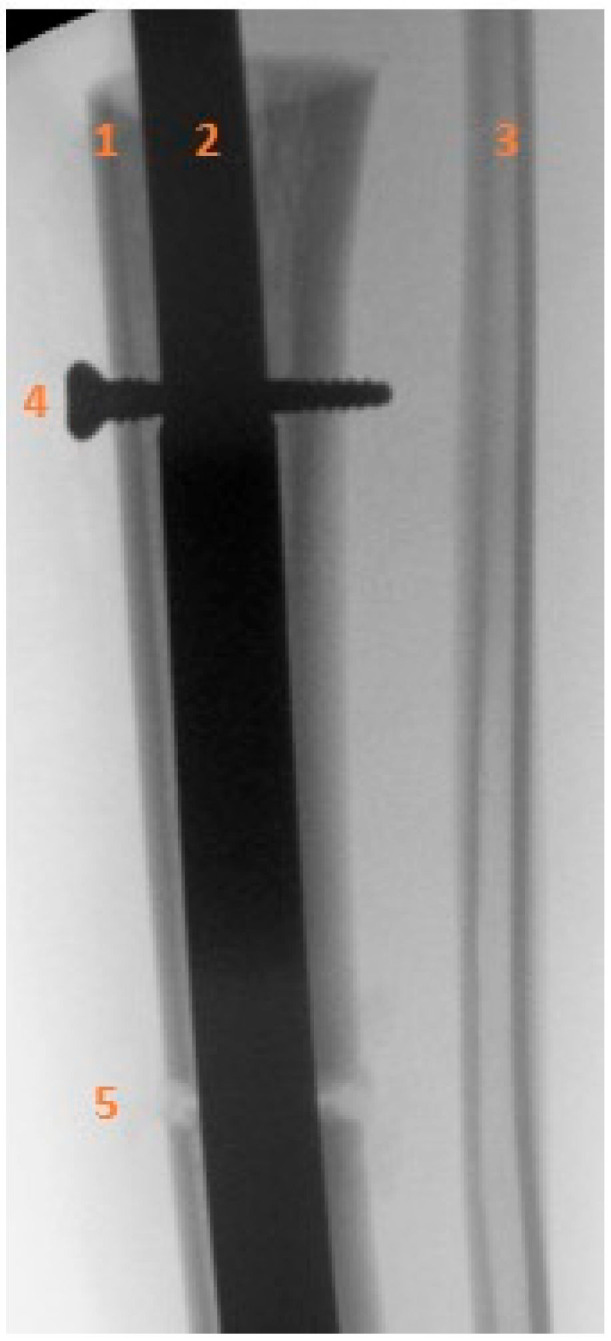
Intraoperative anteroposterior radiograph showing the locking bolt inserted into the transport segment. (1) Proximal tibial diaphysis and transport segment. (2) Intramedullary nail. (3) Intact fibular diaphysis. (4) Locking bolt within the transport segment and internal motor of the intramedullary nail. (5) Distal tibia, osteotomy of the transport segment.
